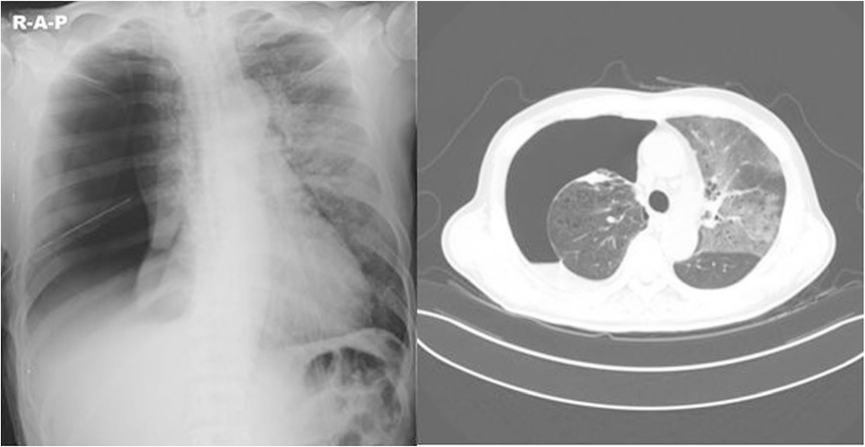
A chest radiograph and chest CT show the right half- collapsed lung, and diffuse and severe infiltrative patterns suggesting reexpansion pulmonary edema in the left whole lung field
